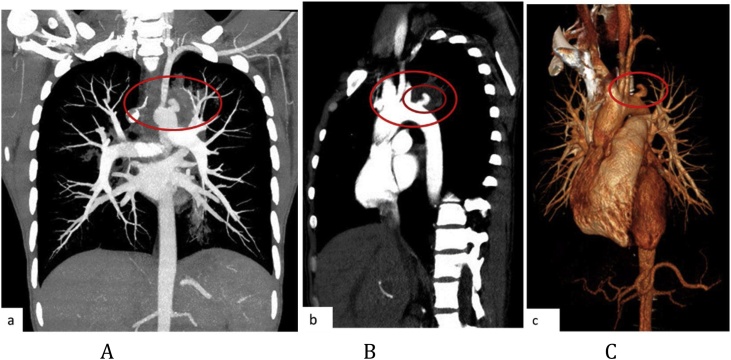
A: CT angiogram of the chest, MIP coronal. B: MIP parasagittal. C: Coronal reconstructed. There is outpouching contrast content reverse ‘S’ shape structure of the aorta lateral to the left subclavian artery with peripheral density as shown by ().
